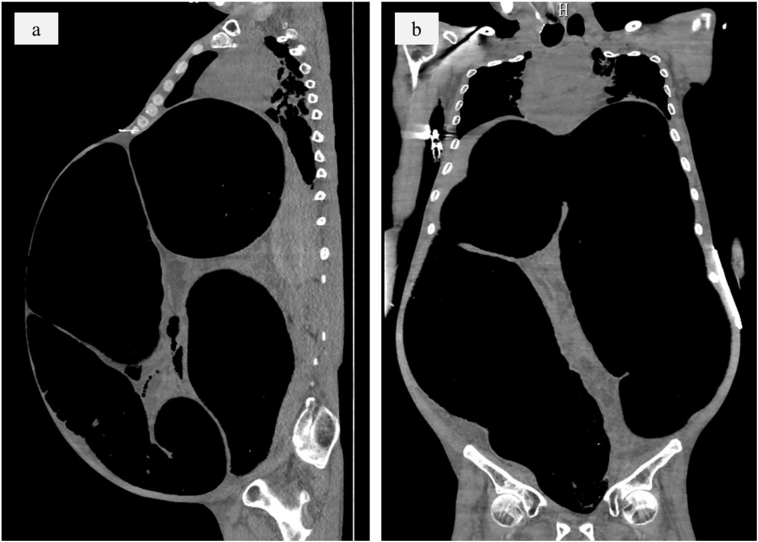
Chest and abdominal computed tomography scan findings. a Sagittal view of the dilated colon filled with gas and feces. b Coronal view revealed an elevated diaphragm which compressed both lungs.
